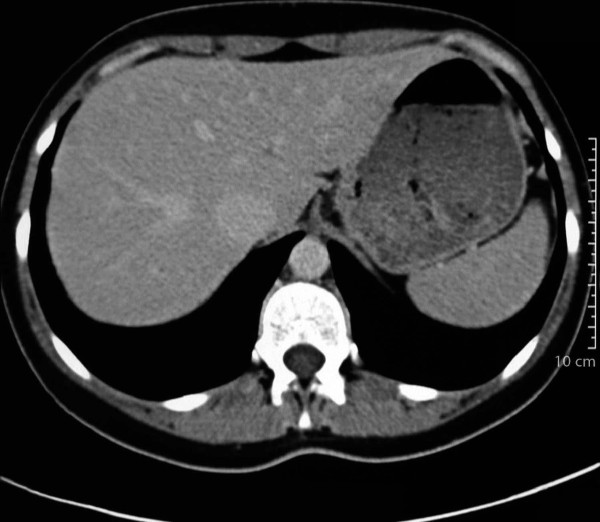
Liver computed tomography scan after trastuzumab maintenance therapy. The lesion has not reappeared.
